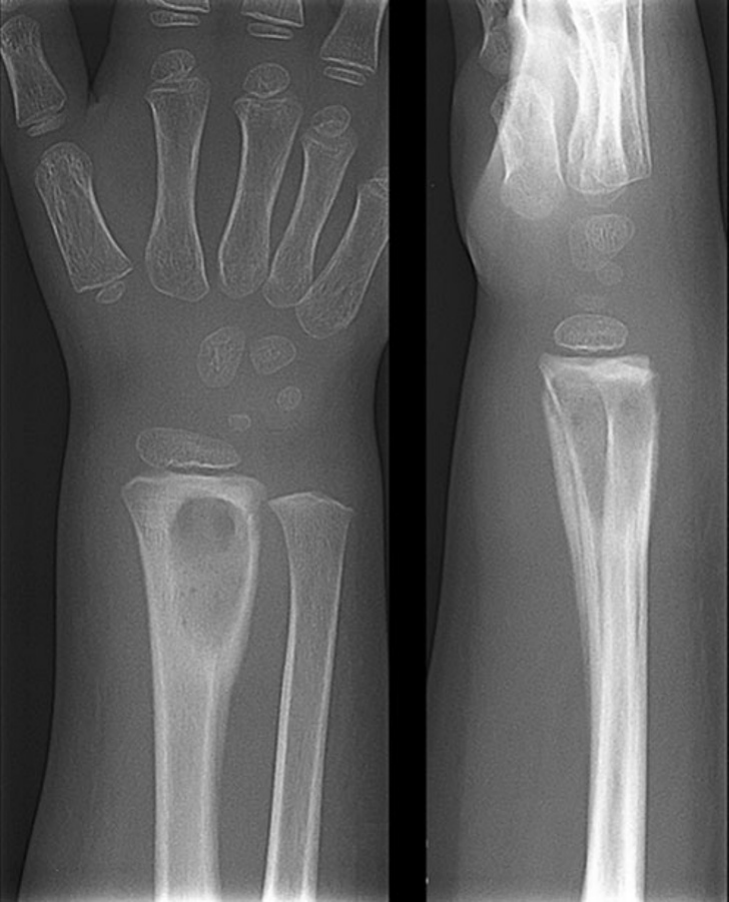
A 3-year-old boy with osteomyelitis of the distal radius. Anterior-posterior and lateral conventional radiographs show a sharply delineated lucent lesion in the distal radius
